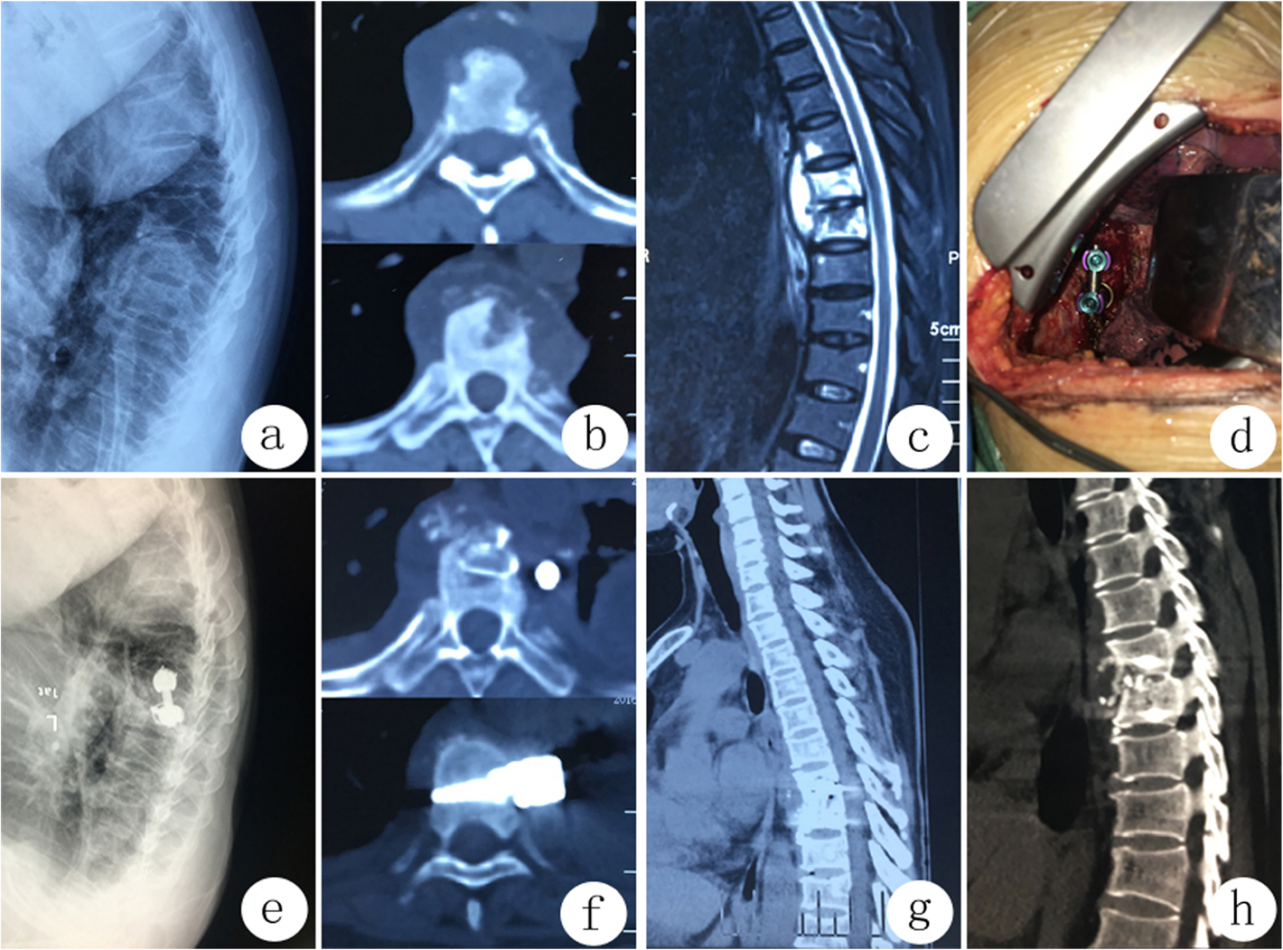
A 49-year-old female patient with T7–8 spinal tuberculosis received anterior transthoracic debridement and fusion. 1a–1c Preoperative images showed paravertebral abscess formation in T7–8 and bone destruction in anterior column T8. 1d Intraoperative findings showed that the screws and titanium rod fixation were in place after interbody grafting with direct visual observation. (1e-g). Postoperative images after two weeks of operation showed that the interbody graft and instrumentation were in place. 1 h Postoperative images after 24 months of operation showed that solid interbody fusion was obtained
